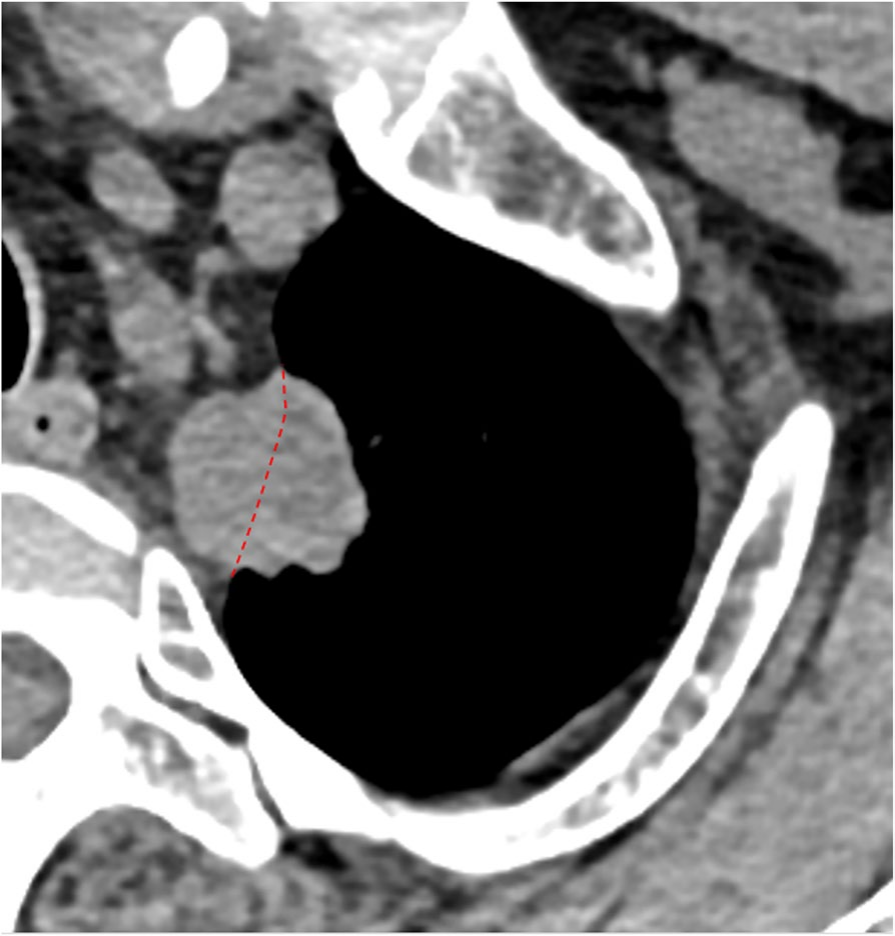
SPAN with type IV of nodule-pleura relationship. A 44-year-old man with left upper lobe SPAN which is confirmed as an invasive adenocarcinoma. On the axial CT image, the lobulated nodule crosses the potential pleural line (dashed line) and extends into extrapleural fat
